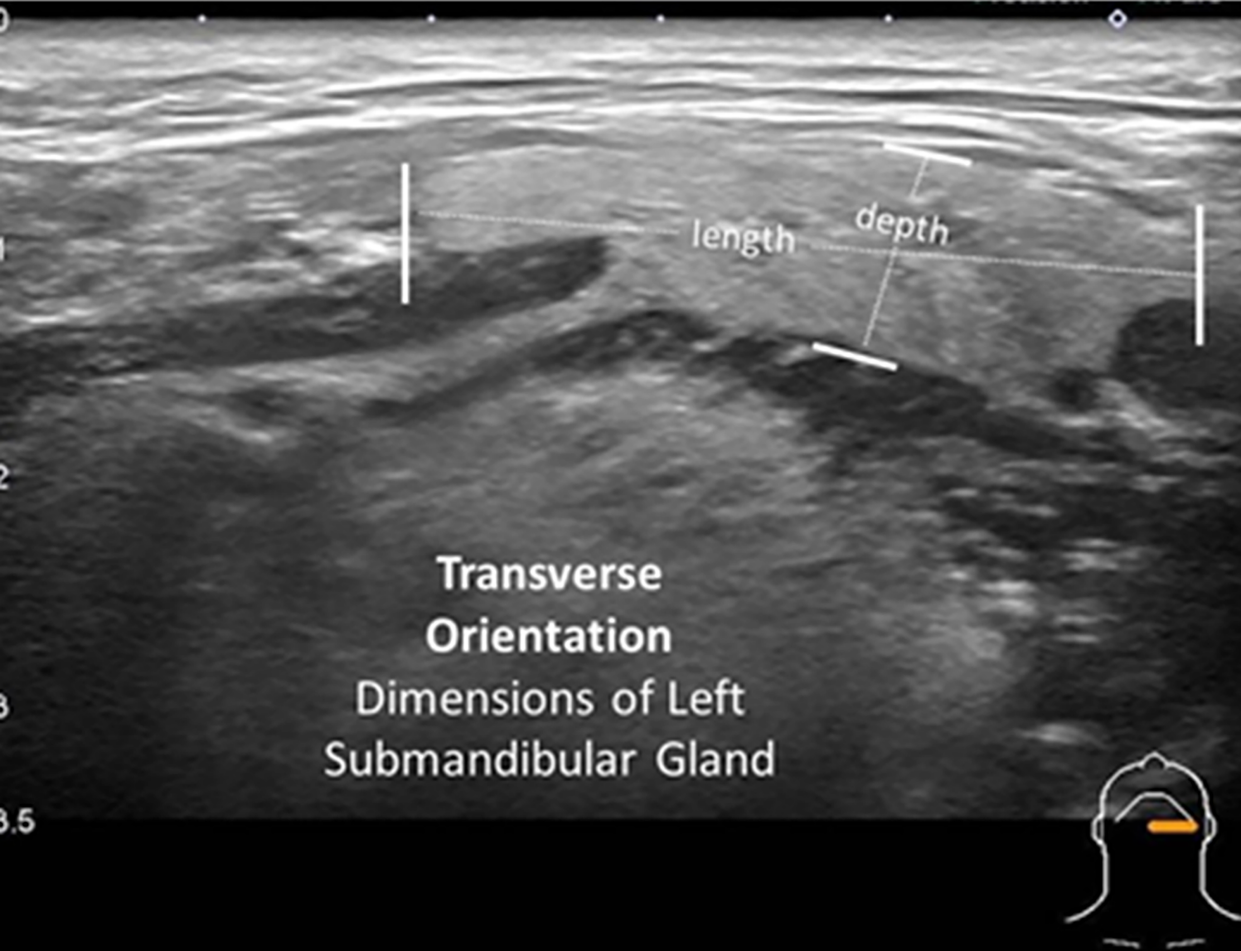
Transverse orientation (with slight obliquity) of the 14–5 MHz linear ultrasound probe identifies measurement of the length and depth of the left submandibular gland.
